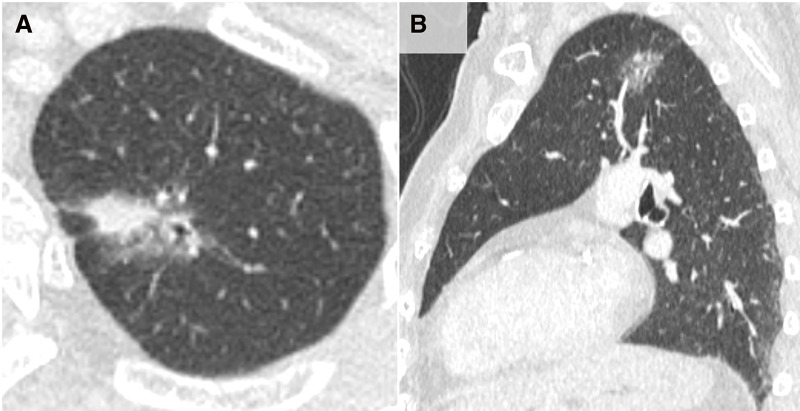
Preoperative computed tomography images. Preoperative computed tomography shows a part-solid ground-glass nodule in the left S^1+2^a involving V^1+2^b (A). The interlobar fissure is not visible (B).
